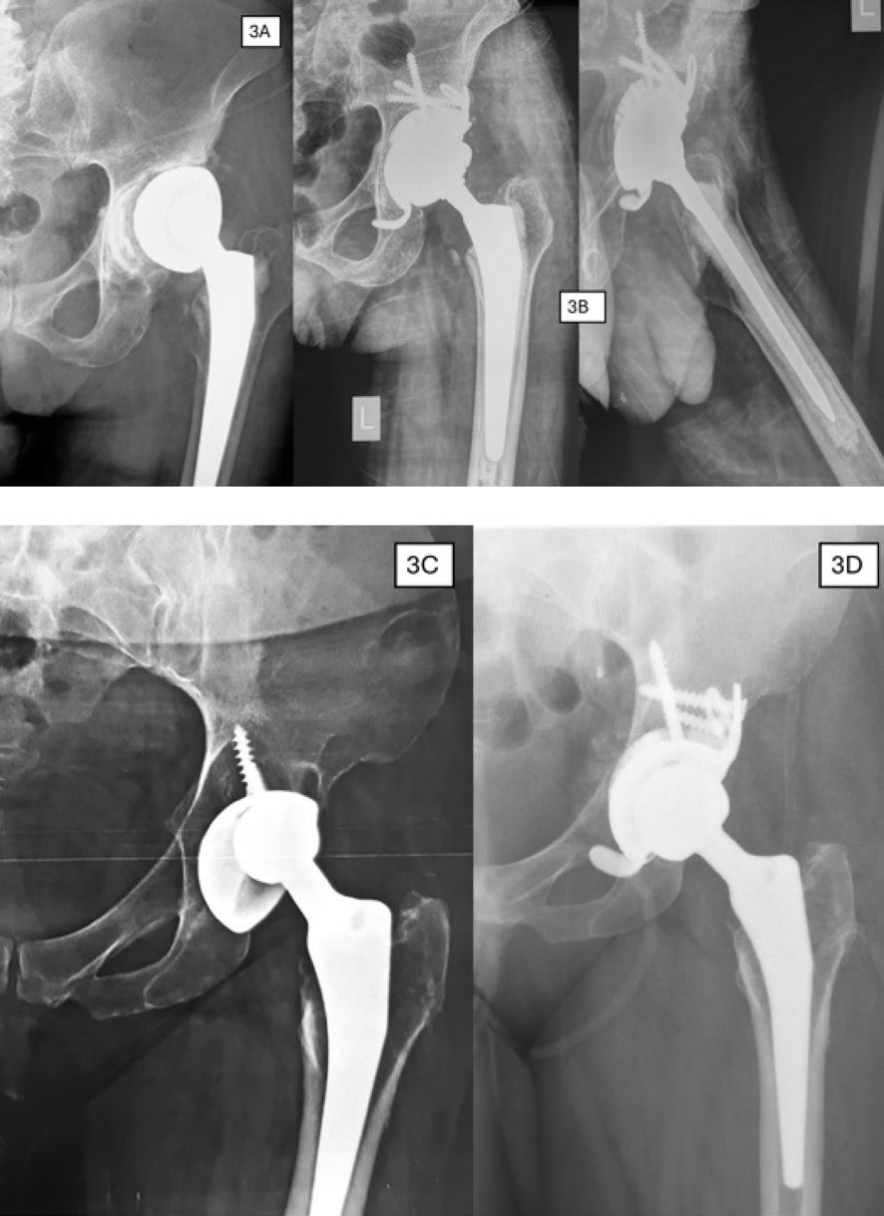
Case examples of Type II Paparosky management: A Preoperative radiograph showing aseptic loosening of cemented dual mobility cup. B Postoperative radiograph at the final follow up visit after Delta TT system implantation. C Preoperative radiograph showing aseptic loosening of cementless acetabular cup. D Postoperative radiograph at the final follow up visit after Delta TT system implantation
